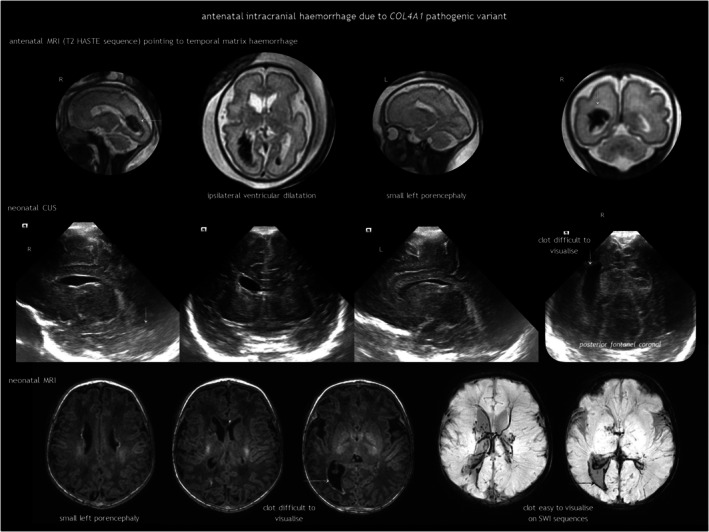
Antenatal intracranial haemorrhage associated with a *COL4A1* pathogenic variant. At 27 weeks of gestation, intraventricular haemorrhage and periventricular haemorrhagic infarction were detected. Magnetic resonance imaging (MRI) at 30 weeks confirmed these findings; a second MRI at 33 weeks showed porencephaly. A de novo *COL4A1* c.4652G>A (p.Cys1551Tyr) variant was identified in heterozygosity. The infant was born at 39 weeks. Abbreviations: CUS, cranial ultrasonography; MRI magnetic resonance imaging; SWI, susceptibility‐weighted imaging.
